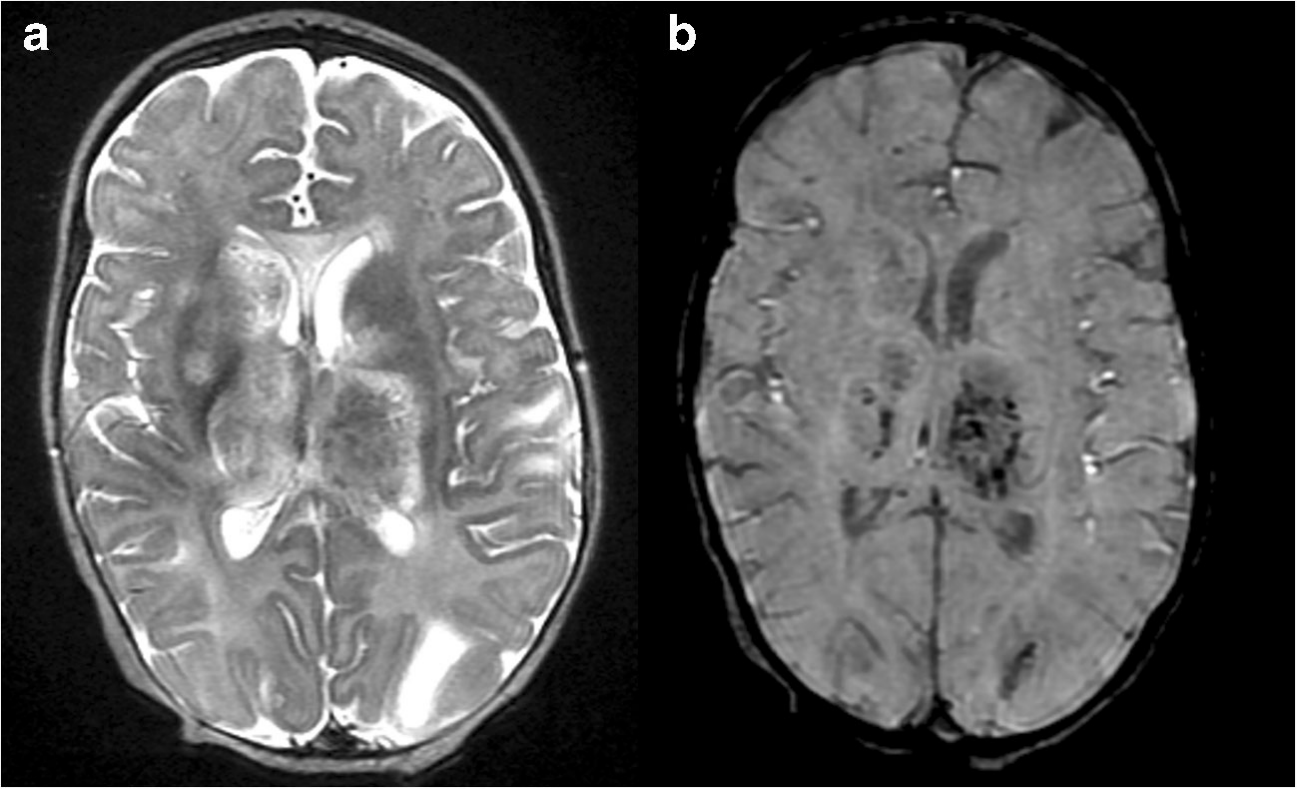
Axial T2-weighted (a) and SWI (b) MR images of the brain at the level of the basal ganglia and thalami. Extensive signal abnormalities are shown in both the basal ganglia and thalami (a) with hemorrhagic foci mainly located in the thalami (b)
